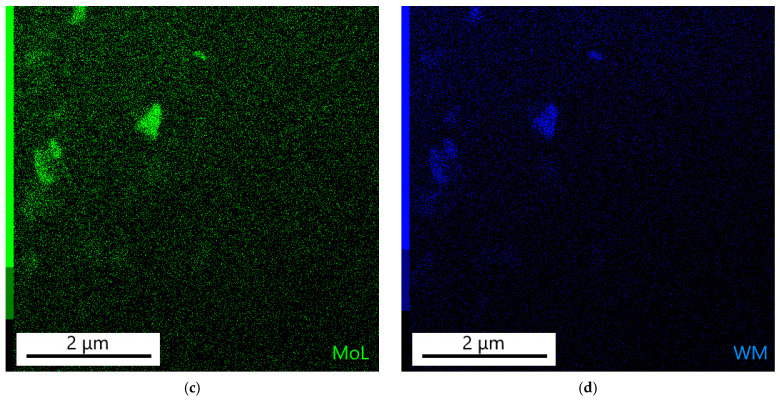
Precipitation in the WM23/P91 fusion zone (mainly M_23_C_6_ and some Mo- and W-rich M_6_X particles): (a) bright field; (b–d) X-ray maps of Cr, Mo and W. Sample B1.
